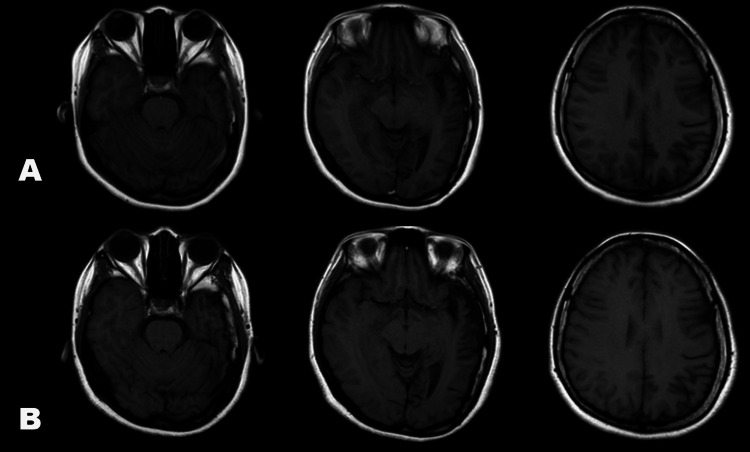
Representative cranial magnetic resonance images (T1 sequences) of the patient. Left cerebral hemiatrophy is apparent in the temporal, occipital, and parietal lobes. Ex vacuo dilatation may be seen in the left occipital area. No significant difference in size were seen between the MRI done in 2018 (Panel A) and 2023 (Panel B).
